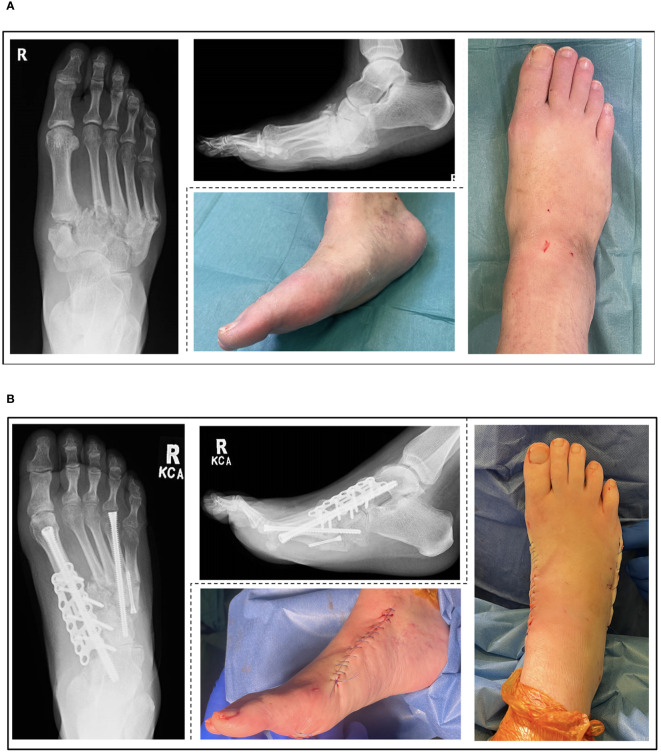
Pre-operative (A) and post-operative (B) radiographic and clinical images of an Eichenholz stage 3 (resolution), Brodsky type 1 (midfoot) Charcot Neuroarthropathy (CNO) treated according to the proposed algorithm.
